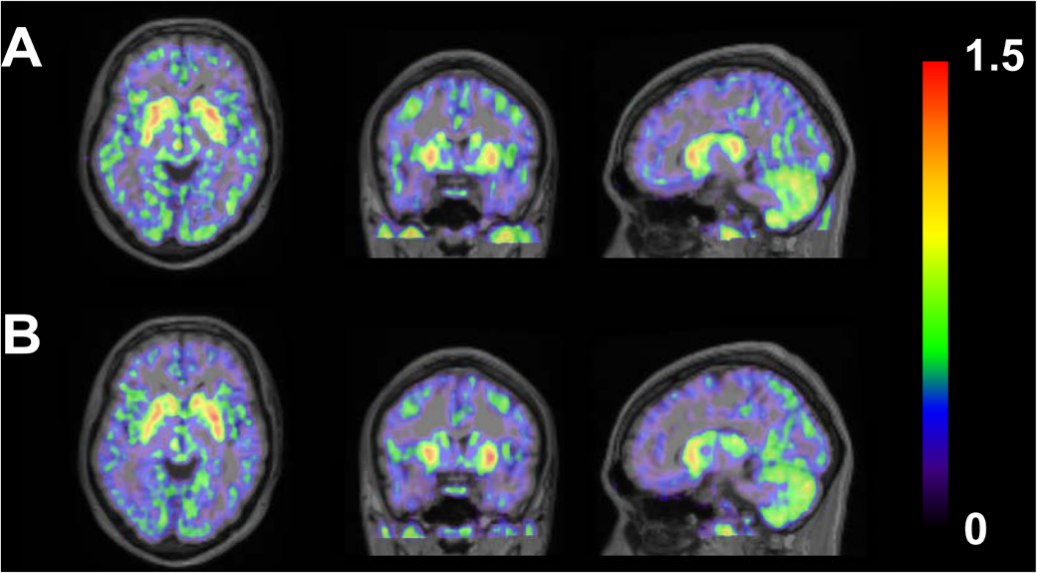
Typical example of parametric image for BP_ND_in (A) test and (B) retest conditions. The centrum semiovale was used as a reference region. The parametric image was fused with the individual subject’s MR image.
